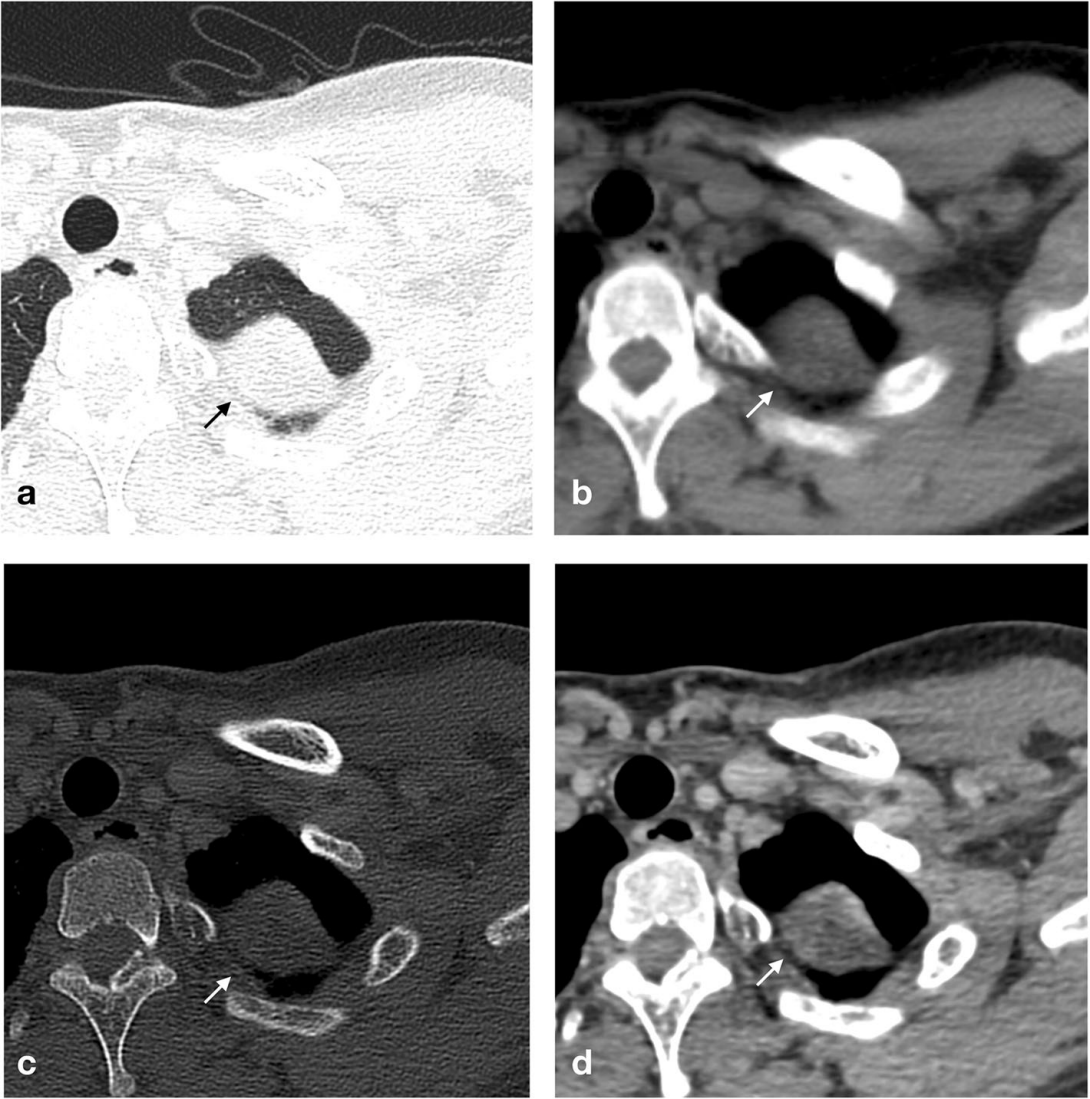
Radiological and pathological findings of case 2 (a-c) Axial CT on the lung, bone and soft tissue windows showed an expansive lesion with destruction and a pathological fracture, as well as an associated soft tissue mass. (d) After contrast administration, enhancement of the mass was demonstrated
